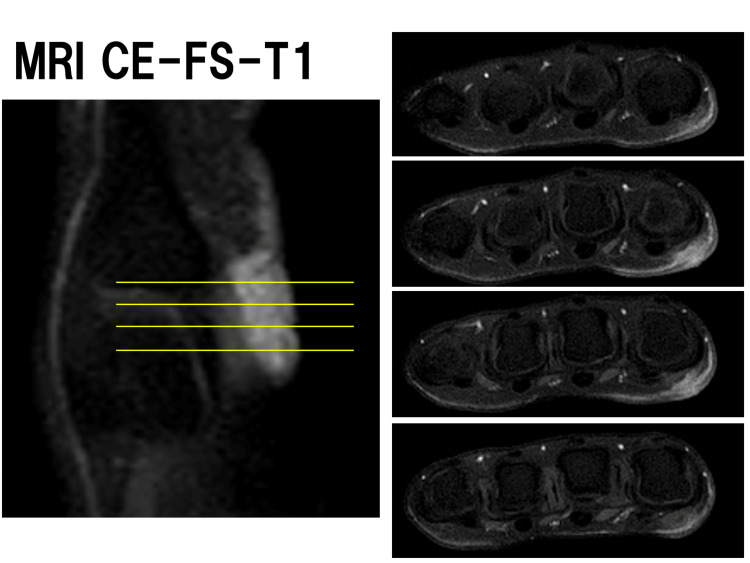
Contrast-enhanced MRI with fat-suppressed T1-weighted images MRI images showed a high-signal area at the index finger MP joint, and the axial view allowed visualization of its extent.
